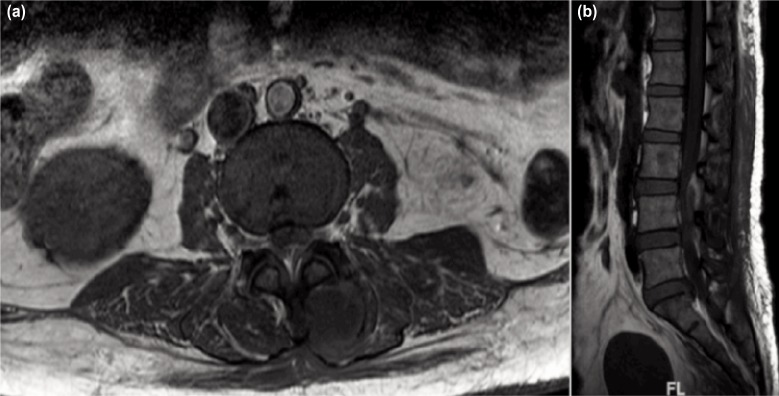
(a) Axial view MRI; The lesion was hypointense on T1-weighted magnetic resonance imaging and was enhancing peripherally on contrast, suggestive of epidural abscess and (b) Sagittal view MRI; The lesion was hypointense on T1-weighted magnetic resonance imaging and was enhancing peripherally on contrast, suggestive of epidural abscess.
